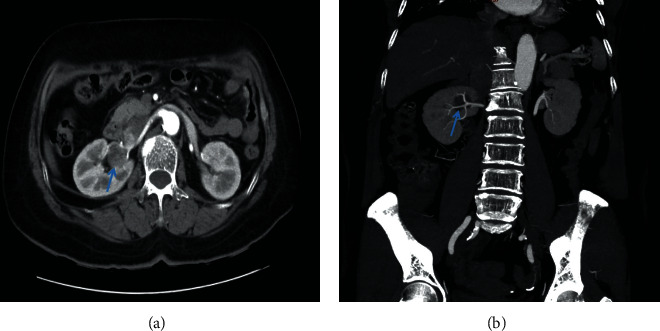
The CT image of a complex renal tumor. (a) CT coronal section. (b) CT transverse section.
